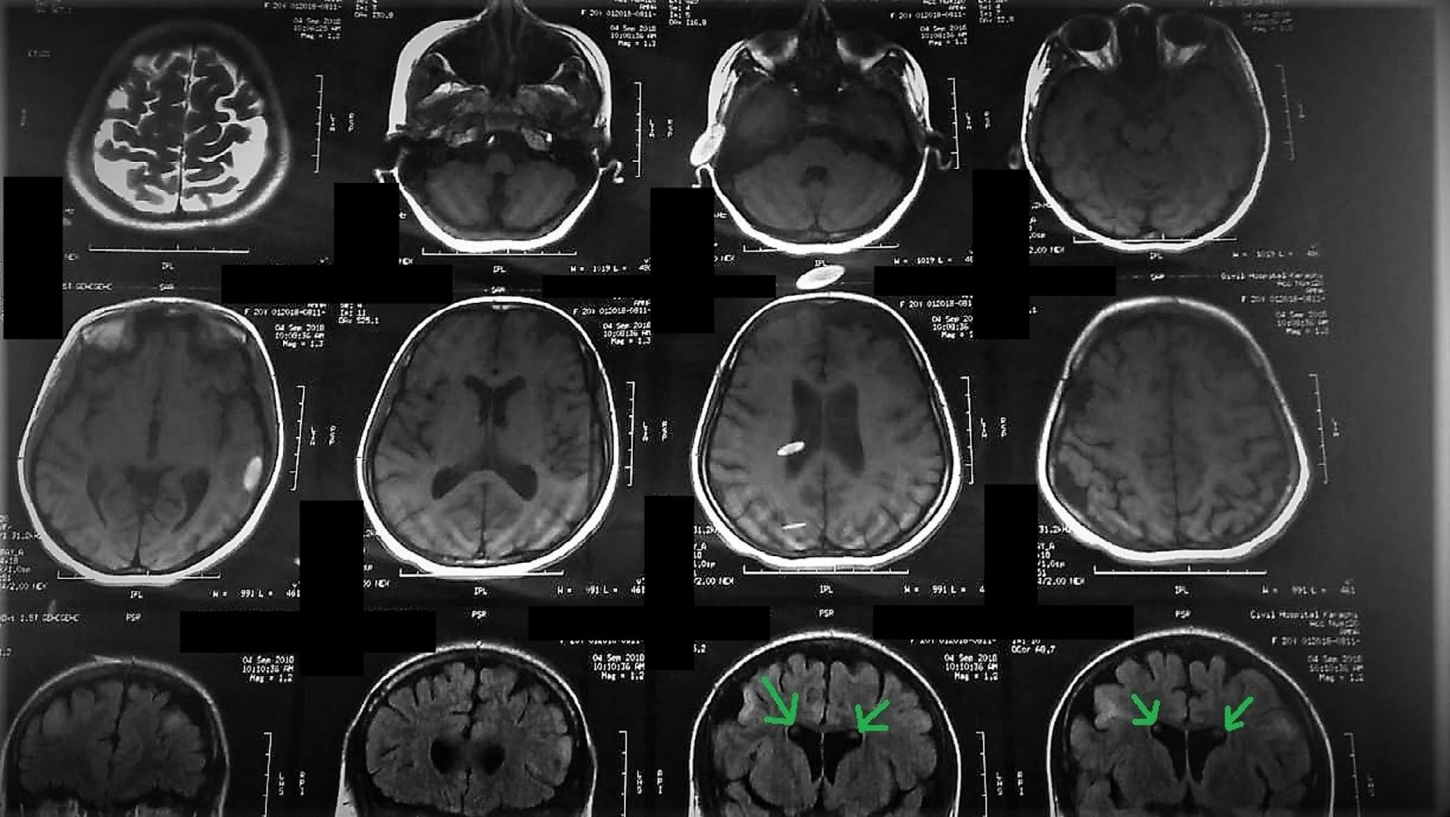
MRI of the brain (axial and coronal planes) shows subependymal nodules (green) are seen returning isohypointense signals onT2 and hyperintense on T1 without showing post‐contrast enhancement
